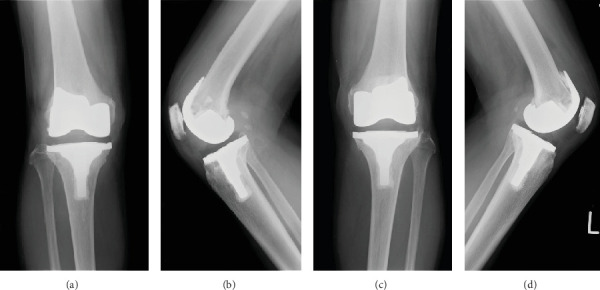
Postoperative plain radiograph after simultaneous bilateral bicruciate-stabilized total knee arthroplasty. (a, b) Right knee and (c, d) left knee. (a) Anteroposterior view, (b) lateral view of the right knee, (c) anteroposterior view, and (d) lateral view of the left knee.
